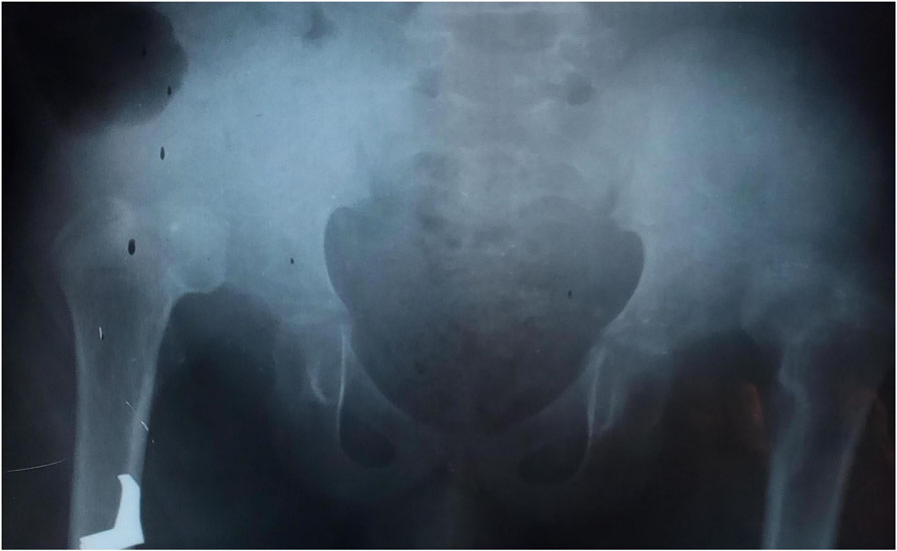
Pelvic X-ray of a 12-year-old boy with bilateral hip dysplasia
